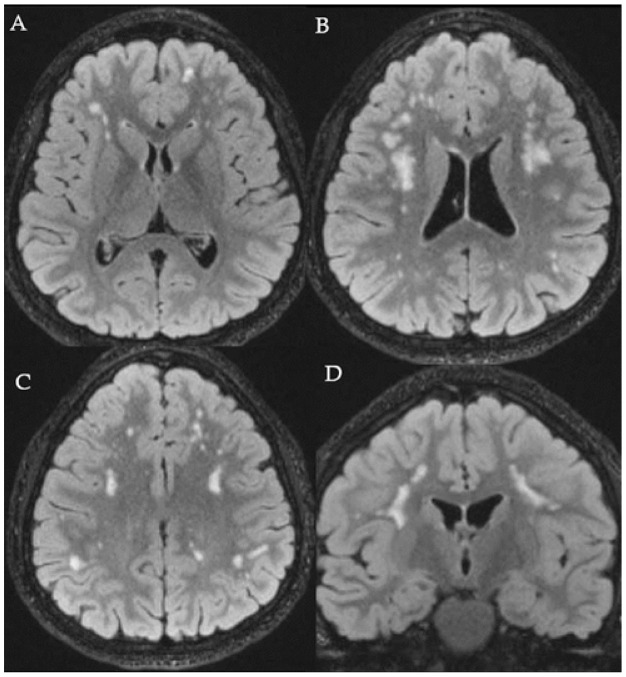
Brain MRI of the patient axial (A–C) and coronal (D) FLAIR images show multifocal and confluent abnormalities in the subcortical white matter of the frontoparietal lobes; stable during the follow-up.
